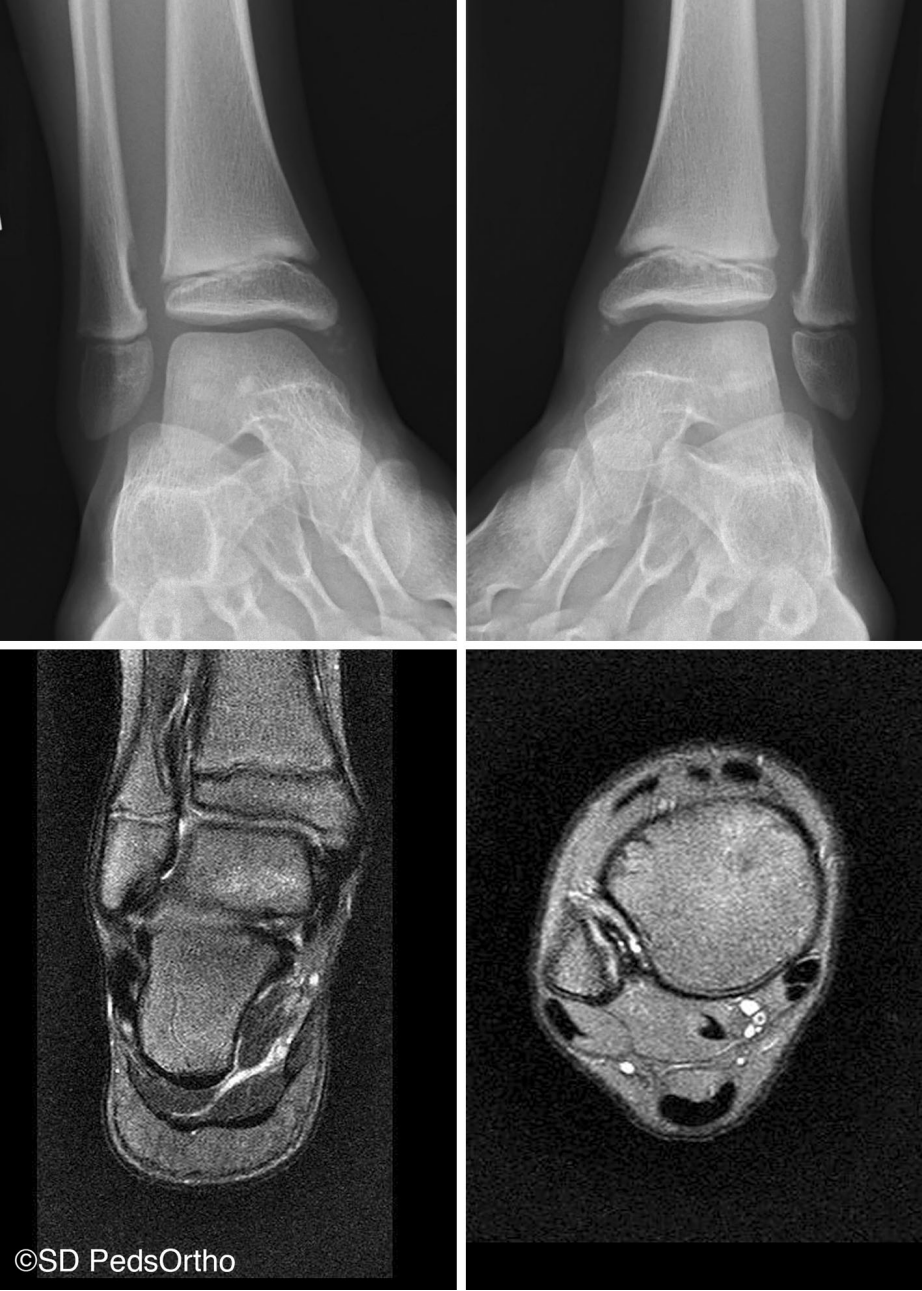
Bilateral distal fibular NOFs, each in communication with the distal extent of the interosseous ligament on MRI
